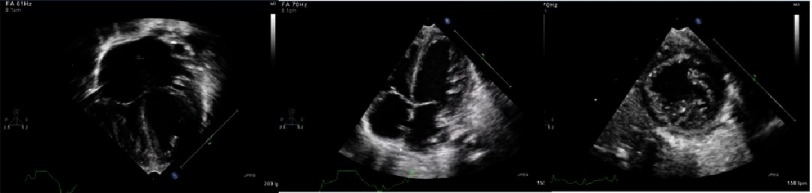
Echocardiography of a newborn diagnosed with Barth syndrome. 1) 4 chamber view shows biventricular hypertrophy, 2) shows mixed non-compaction and LV hypertropphy and 3) short axis view shows postero-apical non compaction.
